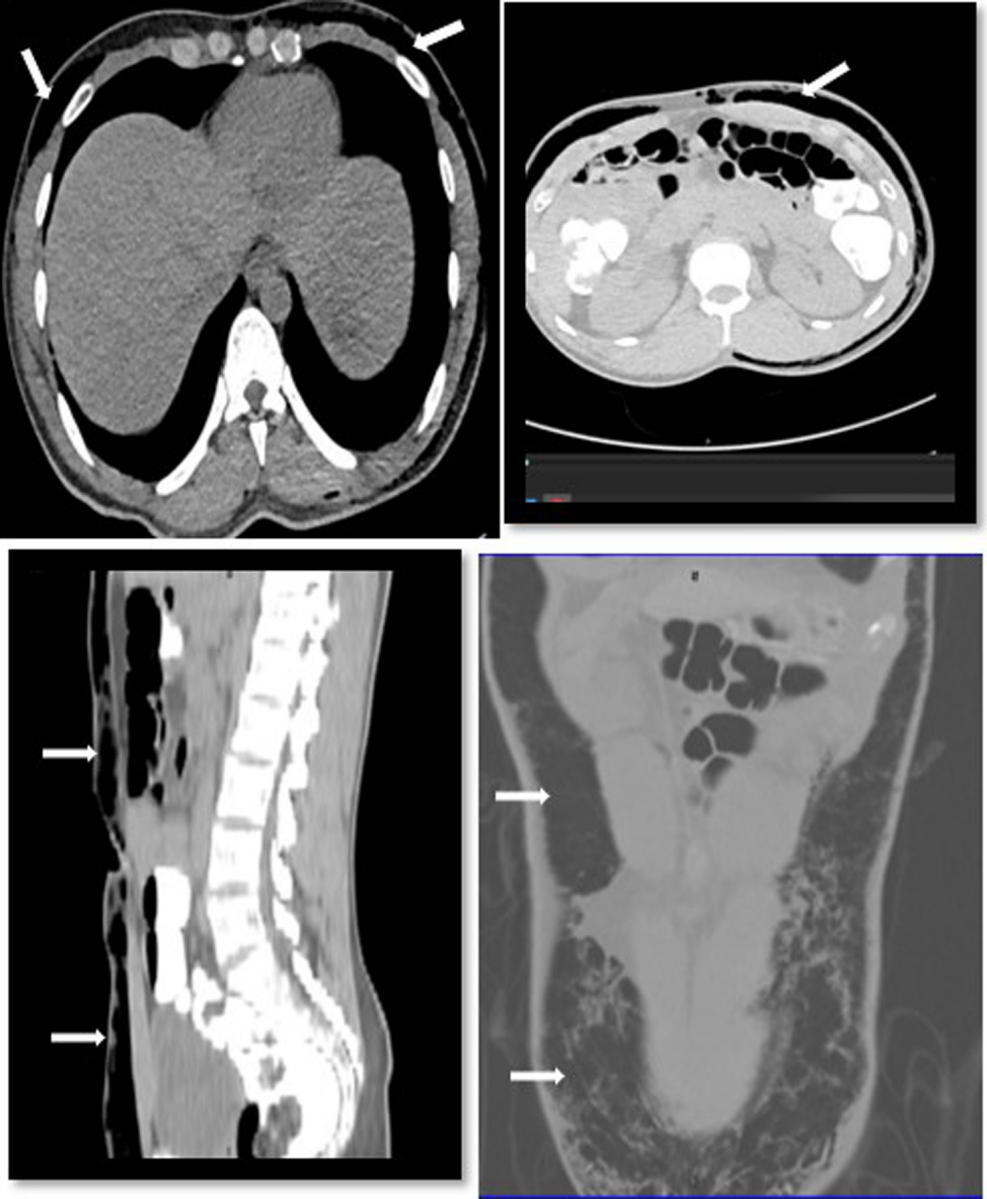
CT scan of chest, abdomen and pelvis showing subcutaneous emphysema.
